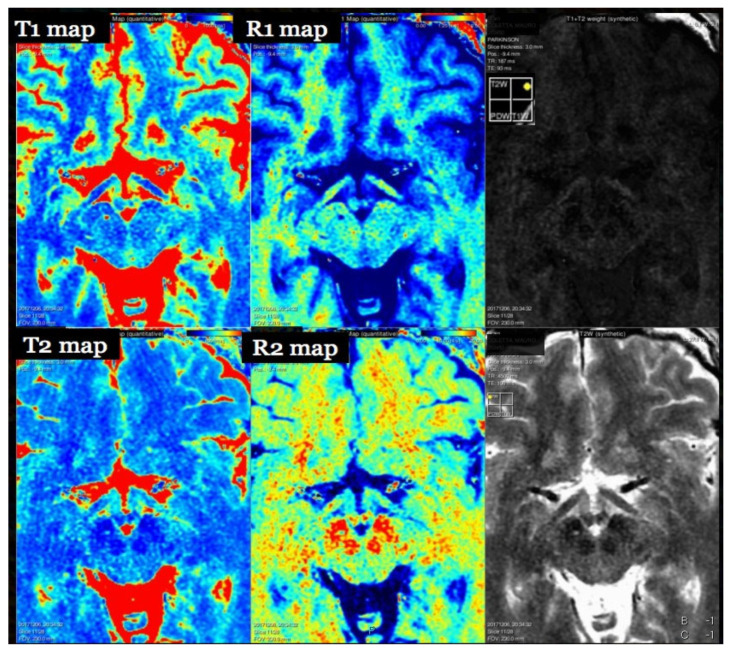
Quantitative parametric maps and synthetic contrasts from a single qMRI acquisition. Representative brain slice showing T1 map (ms), R1 map (s^−1^; R1 = 1/T1), T2 map (ms), and R2 map (s^−1^; R2 = 1/T2) (left and middle panels), alongside synthetic contrast–weighted images reconstructed from the quantitative data (right panels; example T1 + T2-weighted and T2-weighted). As expected, CSF exhibits long T1/T2 (high values on T1/T2 maps; correspondingly low R1/R2), white matter shows shorter T1/T2 (higher R1/R2), and gray matter is intermediate; iron-rich deep nuclei tend to have elevated R2. These maps enable region-wise statistics (e.g., histograms), longitudinal monitoring (ΔT1/ΔT2/R1/R2), and on-the-fly synthesis of conventional contrasts by selecting TE/TR/TI in post-processing. Color scales are arbitrary but consistent across maps for visualization.
